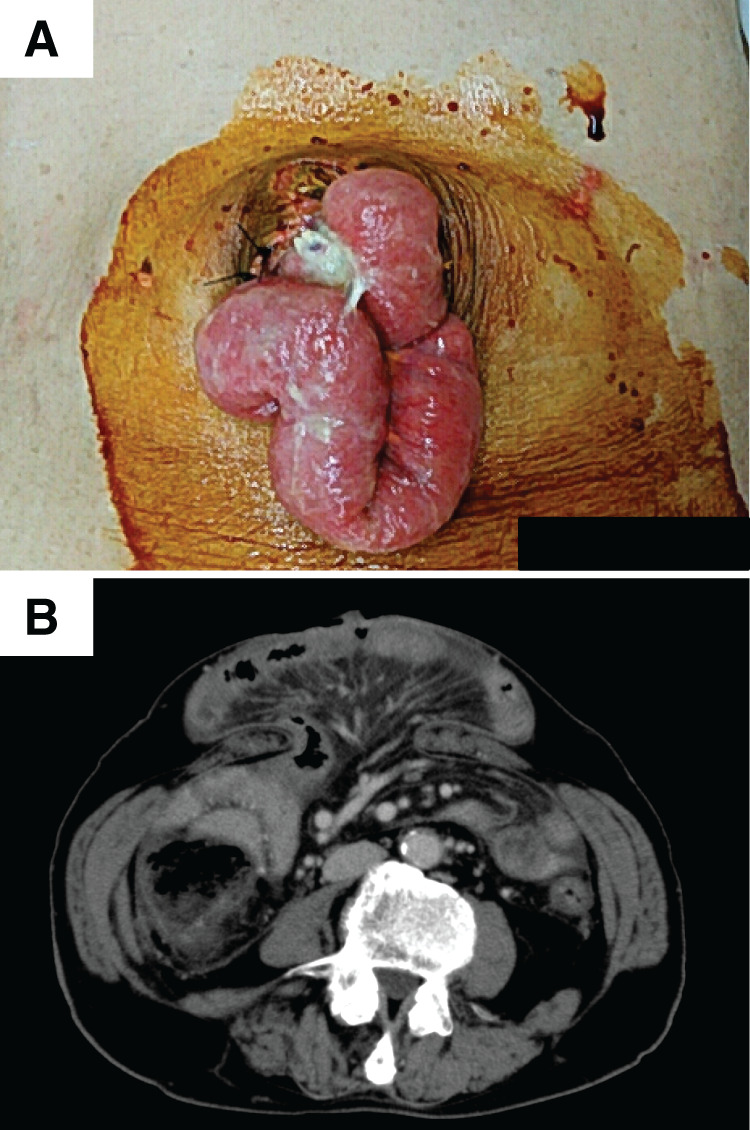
(A) Incisional hernia at the umbilical region appeared, caused by the strong compression from ascites. Skin breakdown at the umbilicus led to intestinal evisceration. (B) Abdominal computed tomography showing incisional hernia at the umbilical region.
